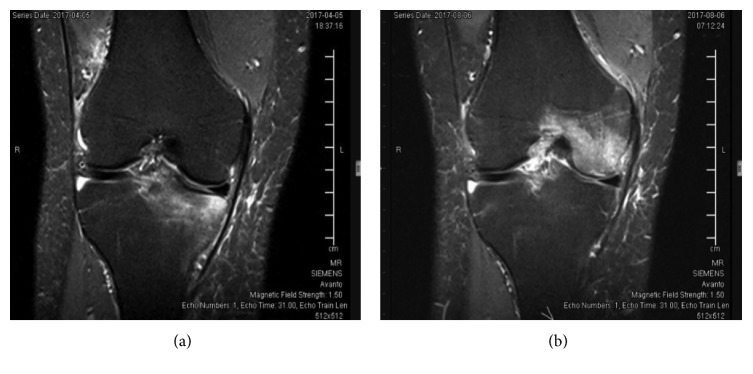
MRI of the left knee (a) in April 2017 and (b) four months later.
